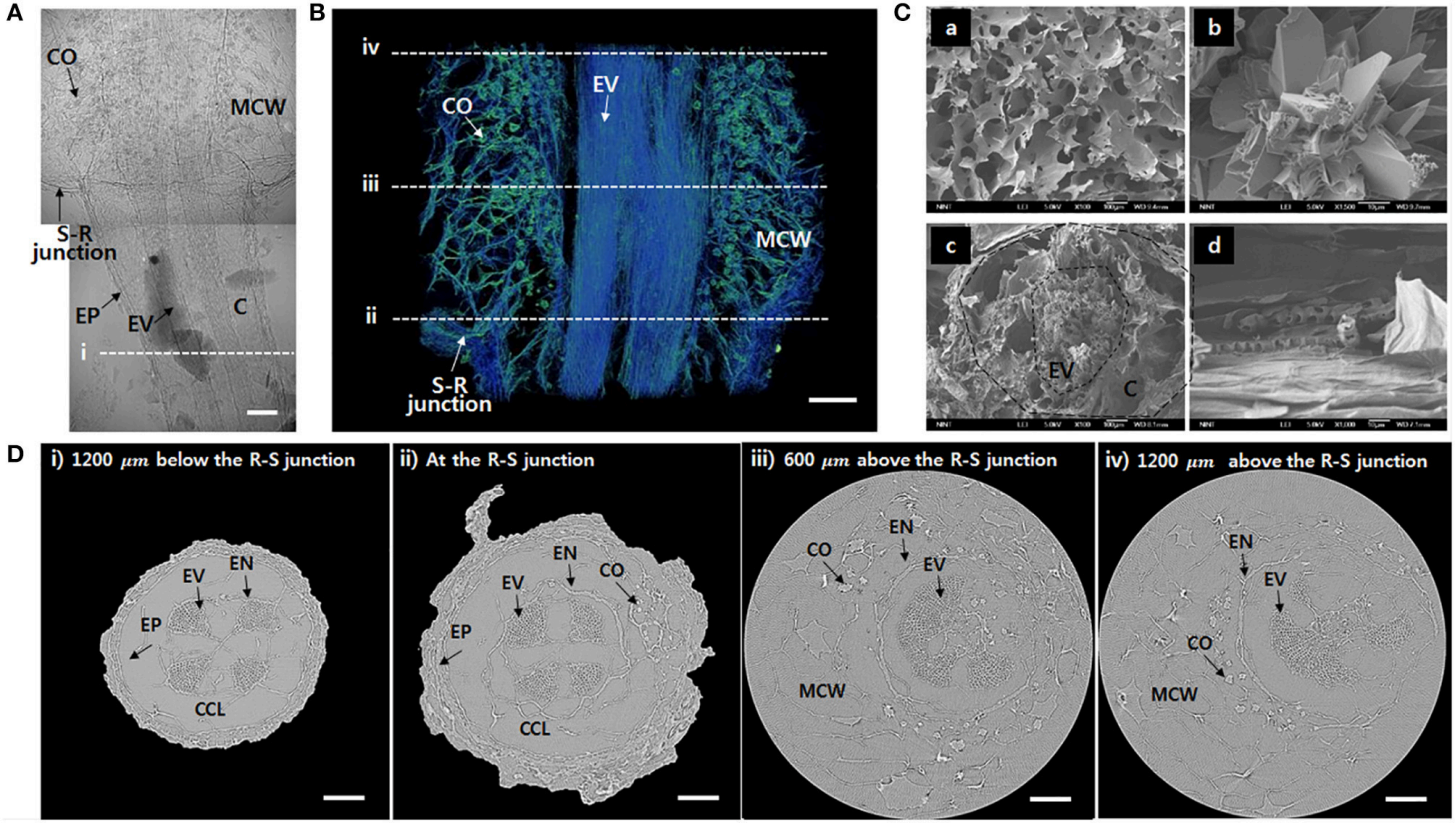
Morphological features of the freeze-dried *O. microdasys* at the R–S junction. (A) Typical 2D X-ray image of the freeze-dried *O. microdasys* at the R–S junction. (B) 3D image of the R–S junction. (C) SEM images of (Ca) mucilage cell wall, (Cb) calcium oxalate inside the stem, (Cc) radial cross-section, and (Cd) axial cross-section of the root. (D) Transverse μ-CT images (Di) at the root 1,200 μm below the R–S junction, (Dii) at the R–S junction, (Diii) 600 μm above the R–S junction, and (Div) 1,200 μm above the R–S junction. These (Di–iv) locations correspond to the dotted lines in (A,B). EP, epidermis; MCW, mucilage cell wall; EN, endodermis; CCL, collapsed cortex lacunae. Scale bar: 200 μm.
